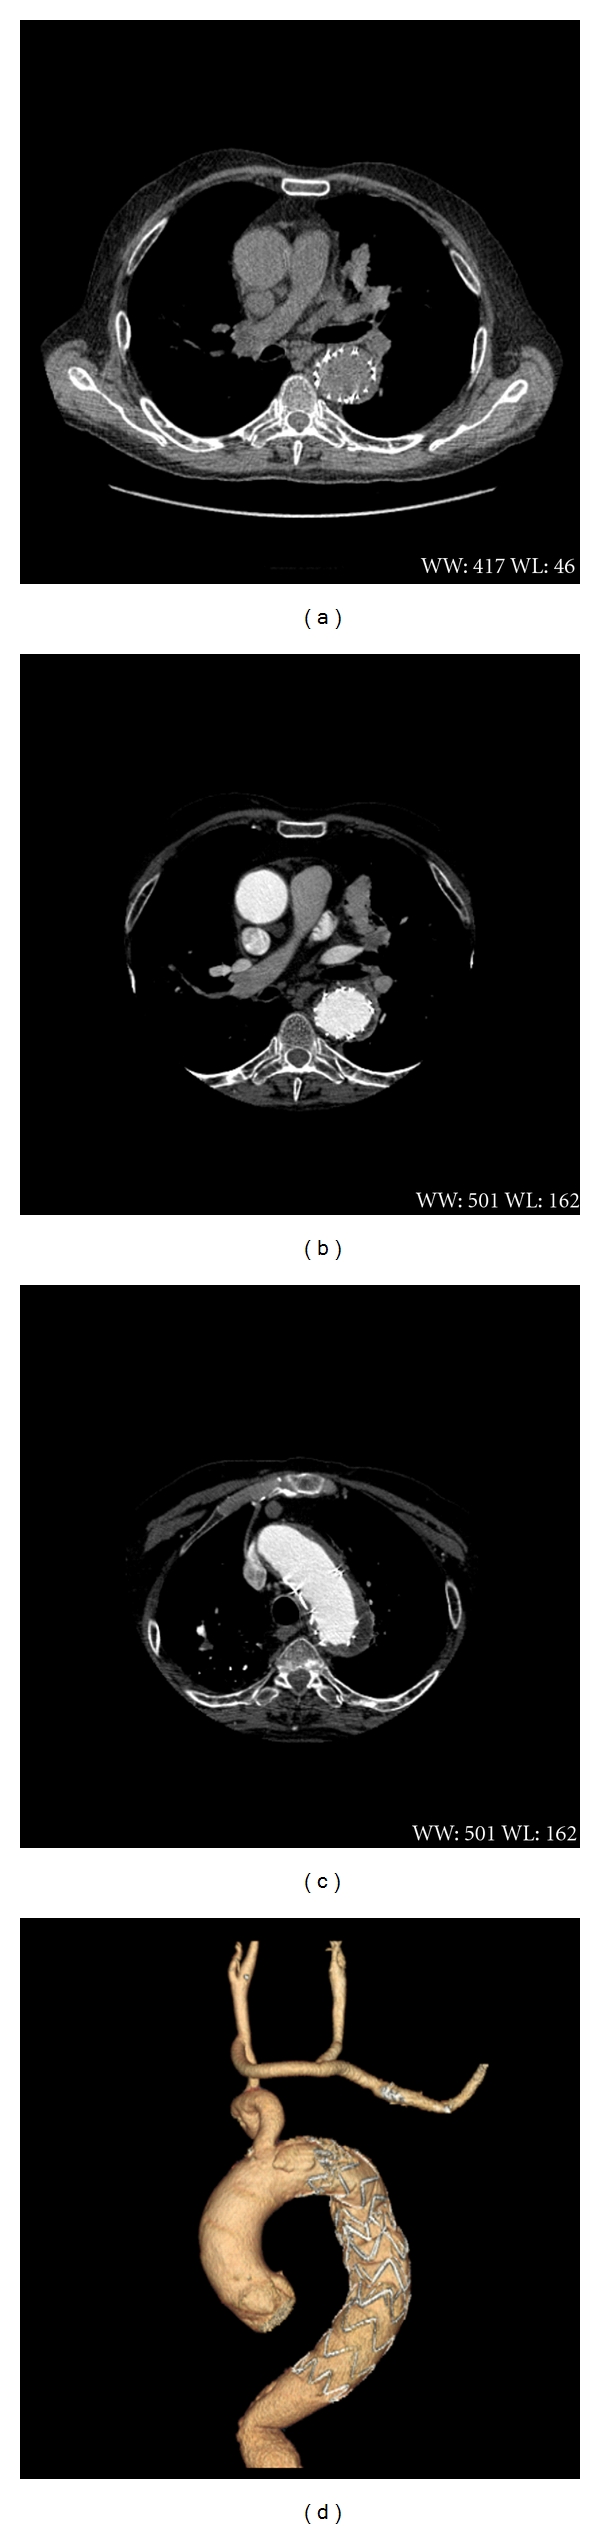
Computed tomographic angiogram at postoperative four weeks later. ((a) and (b)) No observation of intramural hematoma. (c) No observation of true and false lumen. (d) Reconstructed view of the aorta and endograft.
